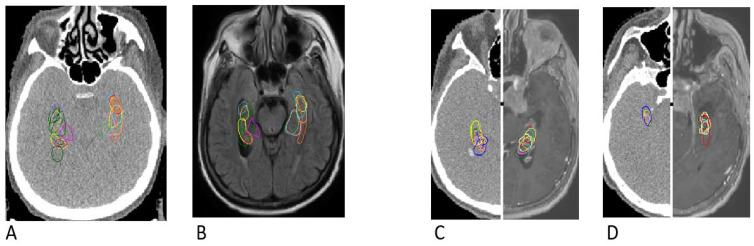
The largest discrepancies in the 1st case of hippocampus contouring (A,B) and the smallest discrepancies in the 9th case of hippocampus contouring (C,D) indicating a positive learning experience by doctors. Differences are visualized on computed tomography images (A, left C and left D) as well as on T1-enhanced MRI sequences (B, right C and right D).
